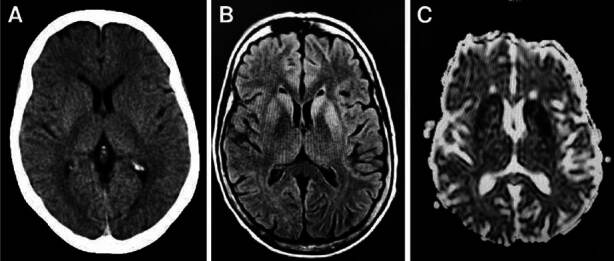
Sporadic Creutzfeldt-Jakob disease. A. Axial non-enhanced head computed tomography image shows no abnormal findings. B. Axial fluid-attenuated inversion recovery magnetic resonance image displays bilateral symmetric high signal intensity of the caudate heads and putamina. C. Axial Apparent Diffusion Coefficient (ADC) map reveals abnormally low diffusion in these regions.
